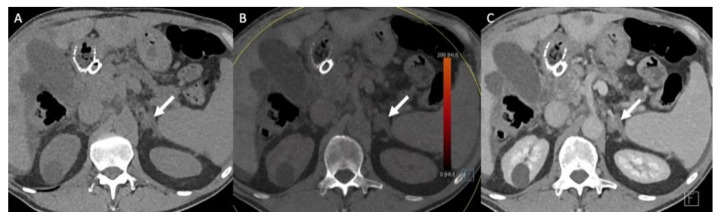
A 70-year-old man with a left adrenal lipid-poor adenoma known for 12 years without any changes in the imaging patterns (arrows). (A) The true unenhanced dual– CT image in the axial plane shows the left adrenal nodule (arrow) with a mean attenuation value of 13 HU. (B) In the virtual unenhanced (i.e., virtual non-contrast) dual-energy CT image in the axial plane, the adenoma (arrow) has a mean attenuation value of 14 HU, which is similar to the mean attenuation value obtained with the true unenhanced CT image. (C) In the venous phase CT image, the adrenal adenoma (arrow) has a mean attenuation value of 59 HU.
